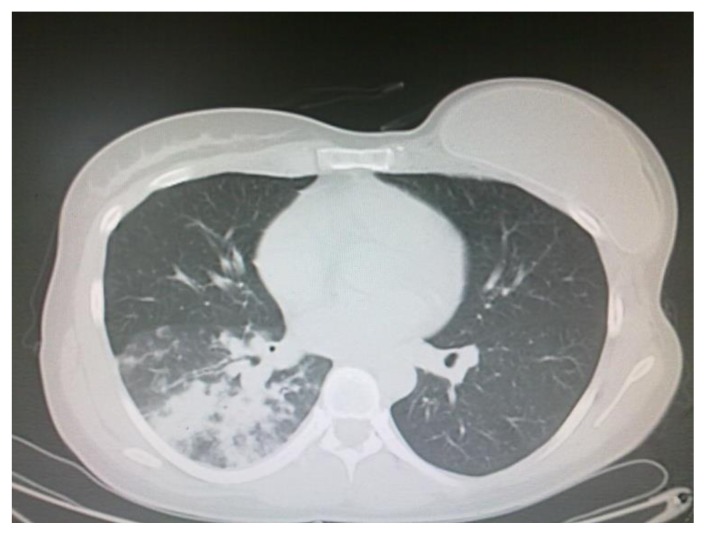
CT Scan at diagnosis of AML: bilateral basal parenchymal consolidation with a consensual right pleural effusion.
